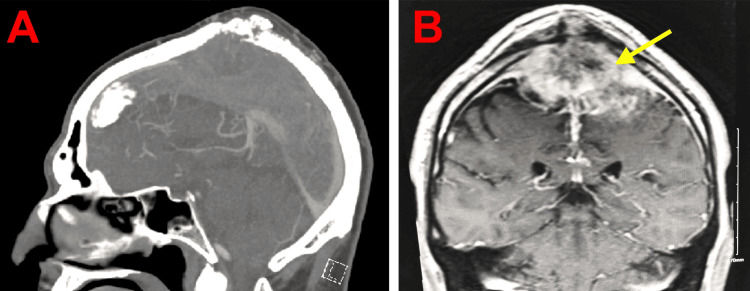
Neuroimaging of large meningioma involving the superior sagittal sinus (A) Non-contrast CT of the head showing a stable, large posterior vertex meningioma with no acute ischemia. (B) Brain MRI showing homogeneous enhancement, diffusion restriction, and superior sagittal sinus encasement CT, computed tomography; MRI, magnetic resonance imaging
